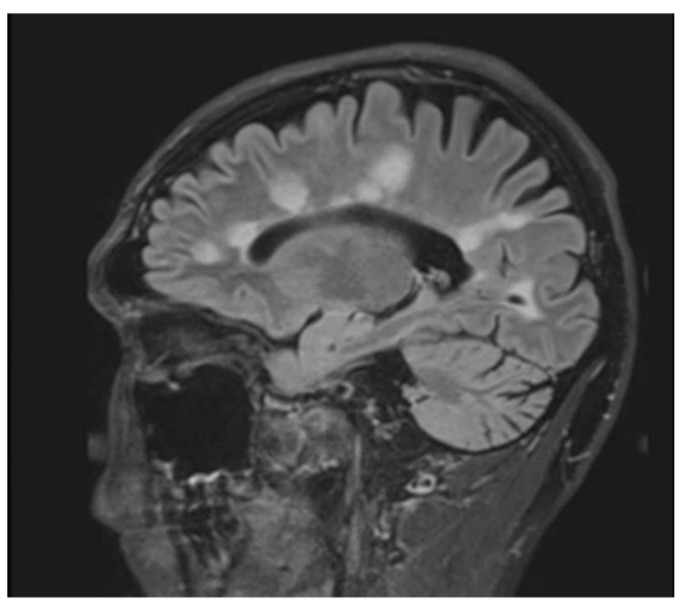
Sagittal fluid attenuated inversion recovery (FLAIR) image shows lesions perpendicular to lateral ventricle giving classic finger-like appearance, Dawson’s fingers.
